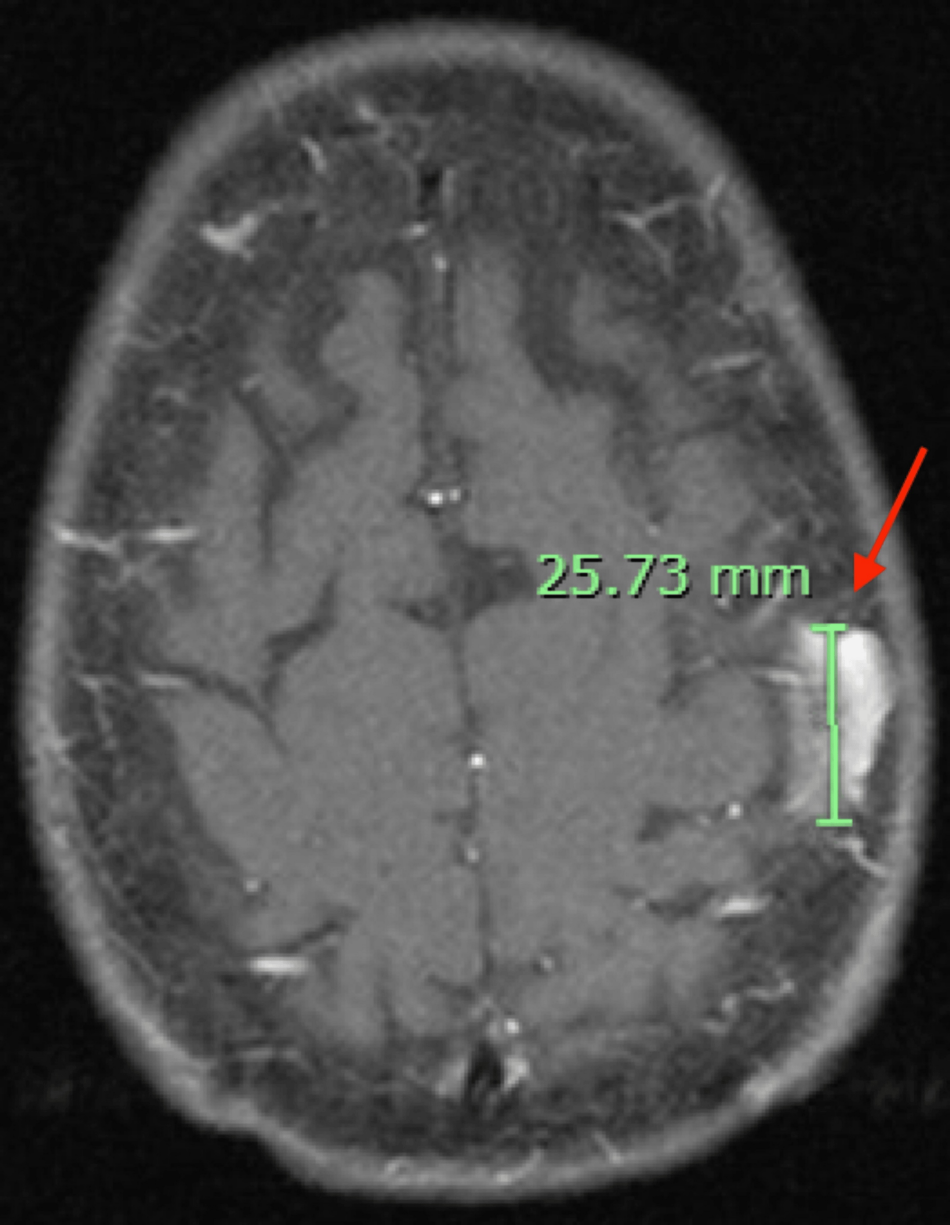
A computed tomography (CT) scan of the head demonstrating the left parietal skull mass extending from the inner table to the outer table calvarium, measuring 25.73 mm in the greatest length, as indicated by the red arrow. No intracranial extension is seen. No metastatic disease to the brain parenchyma is appreciated.
